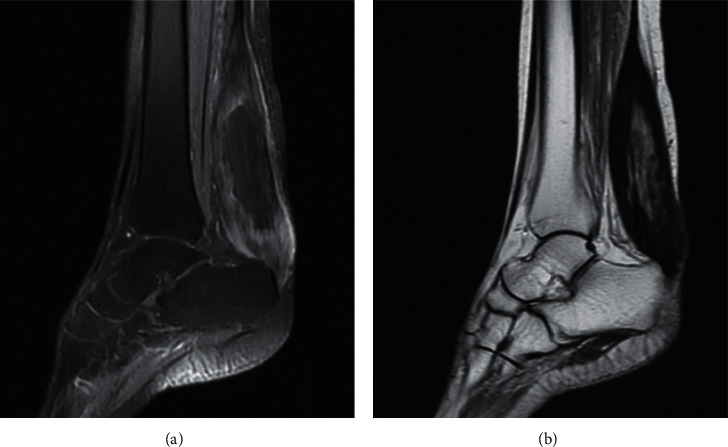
Sagittal images of the ankle MRI showing diffuse thickening of the Achilles tendon hypointense in T1 (a) and heterogeneous hypersignal in T2 (b).
